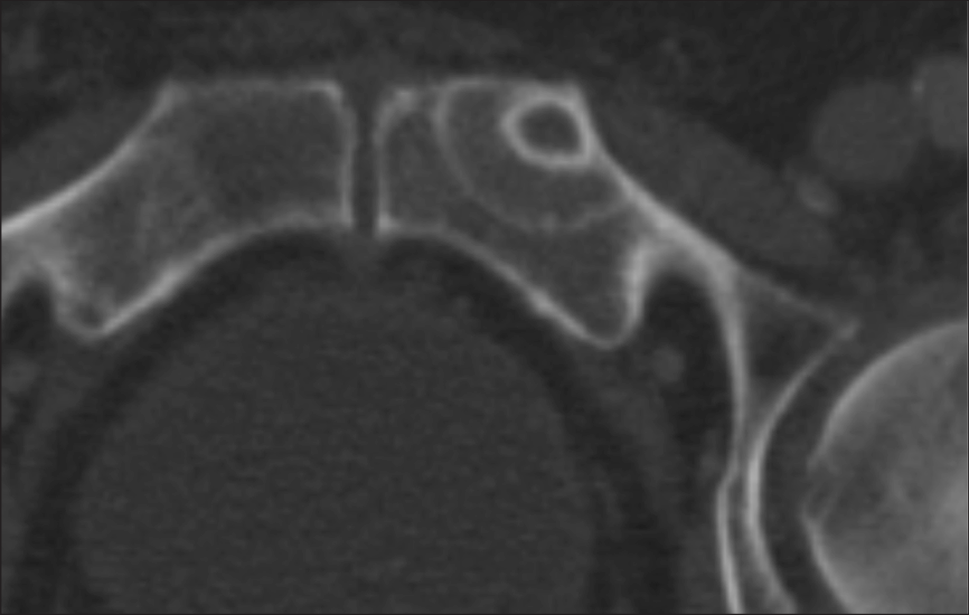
CT image of the same lesion as in Figure 1 after 7 weeks. Note the appearance of a halo with ground glass appearance around the initial lesion with a second thinner sclerotic margin giving it a ‘doughnut’ appearance.
